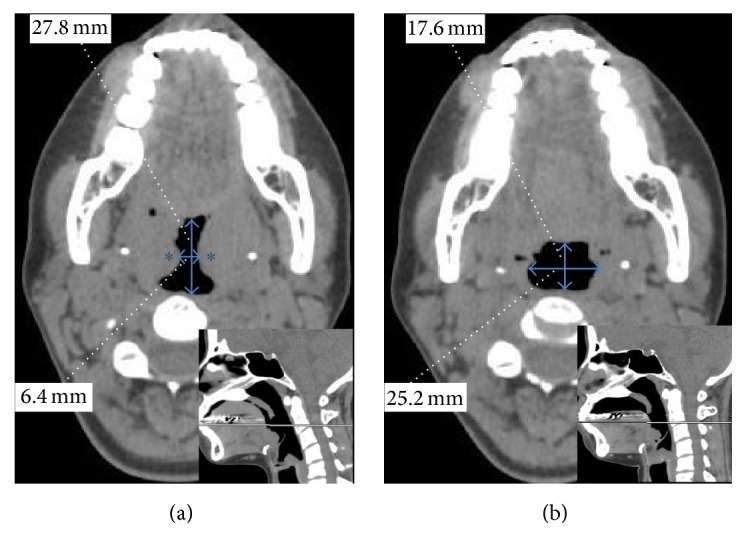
Axial-view computed tomography images from patient 1. (a) The narrowing of glossopharynx (blue double-headed arrows) evident before uvulopalatopharyngoplasty (UPPP) was caused by the impression of large tonsils (asterisk). (b) The narrowing was enlarged transversely; however, the glossopharynx became narrower in the anteroposterior direction after UPPP (indicated by the number). The lines on midsagittal view indicate the level of the axial-view.
